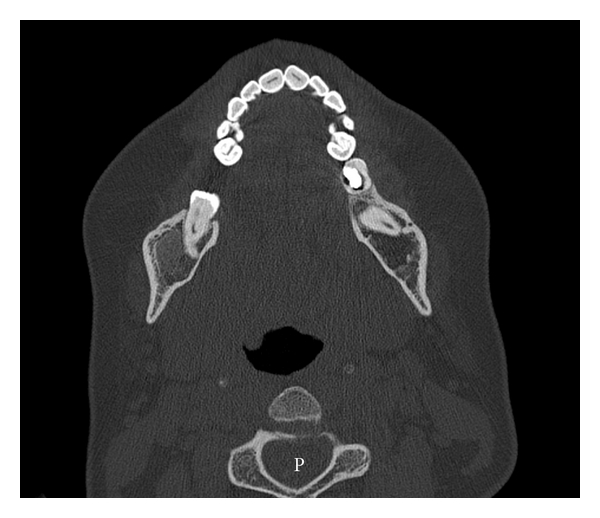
Axial CT scan showing significant regression of the intraosseous lesion with thick and preserved cortical bone at the angle and ramus of the mandible.
